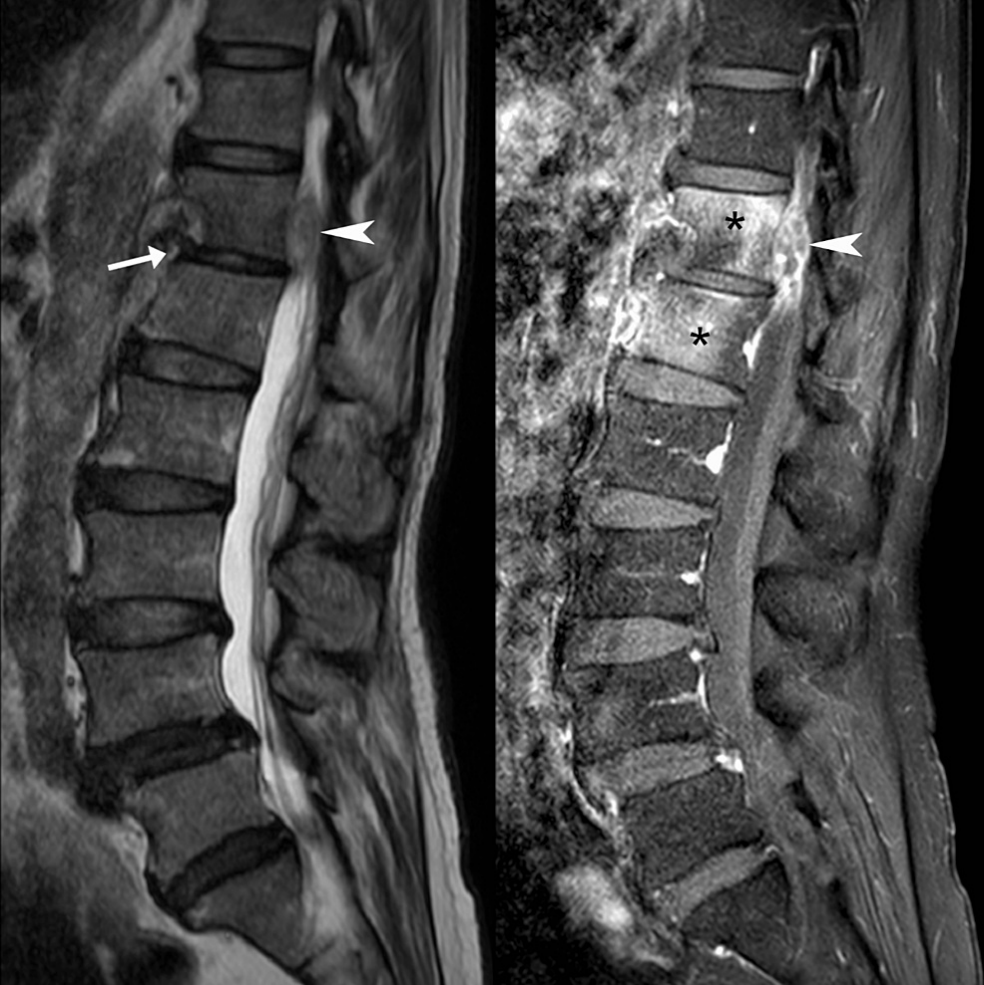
T2-weighted (A) and T1-weighted post-contrast (B) sagittal lumbar magnetic resonance imaging Several signs of pyogenic spinal infection were evident, including a reduction in the T12/L1 disc space (arrow), heterogeneously contrast-enhancing anterior epidural fluid collection, consistent with an epidural phlegmon (arrowhead), and contrast enhancement of the T12 and L1 vertebral bodies (asterisks *), consistent with vertebral osteomyelitis.
